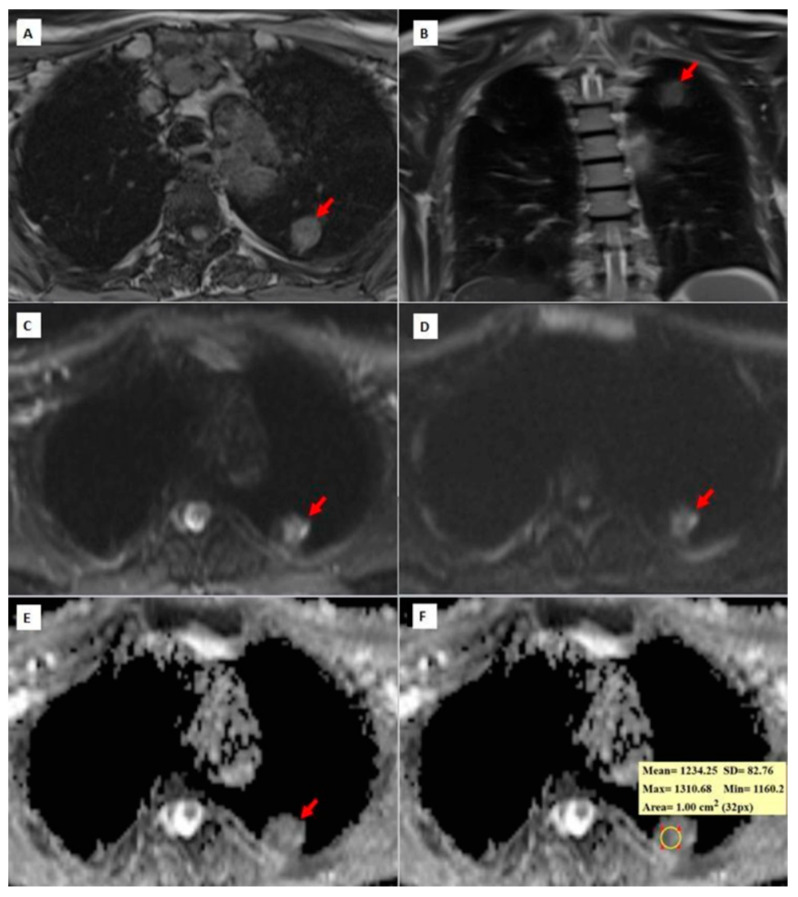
A 62-year-old patient diagnosed with grade 2 squamous cell carcinoma. (A) An axial T1W image showing an isointense, round-shaped lung nodule with a slightly spiculated extension towards the pleura in the upper lobe of the left lung. (B) A coronal T2W MRI image showing an isointense left upper lobe lung nodule (red arrow); (C,D) b = 0 and b = 800 DW images, respectively. In the b = 0 DW images, the nodule has a heterogeneous internal structure and there is a more hyperintense area on the left side of the lesion. In the b = 800 DW images, it is seen that the signal of the lesion decreases slightly and the left side remains more hyperintense than the lesion. (E,F) ADC maps showing a hypointense nodule and a mean ADC value of 1234.25 × 10^−6^ mm^2^/s. While placing the ROI for measurement on the ADC maps, the asymmetric signal area on the left side of the lesion was excluded in the DW images in order to prevent the DW parameters from being affected by non-tumor areas, such as necrosis, abscess, etc., within the tumor.
